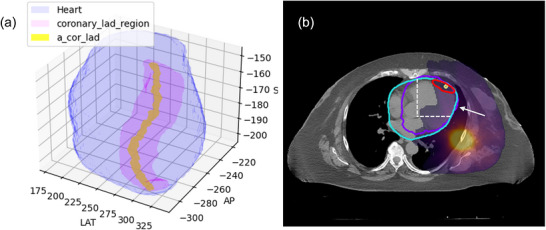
Region definition and registration approach. (A) The LAD and LAD_REGION visually displayed for one patient. (B) The dose distribution and purple heart contour are associated with a lung SBRT patient, whereas the displayed CT, blue heart contour, red LAD_REGION and yellow LAD contours are associated with a breast cancer patient. The registration is focused axially on the left anterior portion of the heart as indicated by the white dashed lines and arrow to preserve the dosimetric impact of the heart lung interface.
